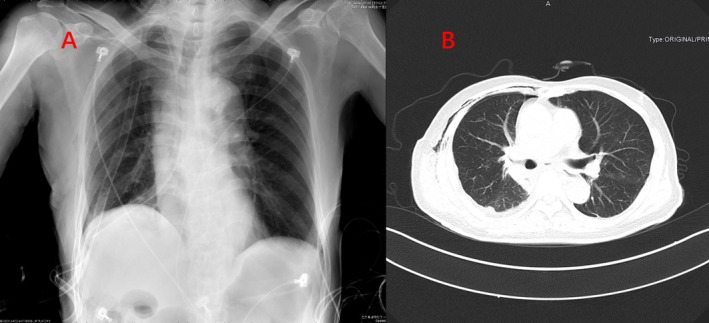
Postoperative X‐ray and computed tomography images of the octogenarian patient. (A) X‐ray image. (B) Computed tomography image.
